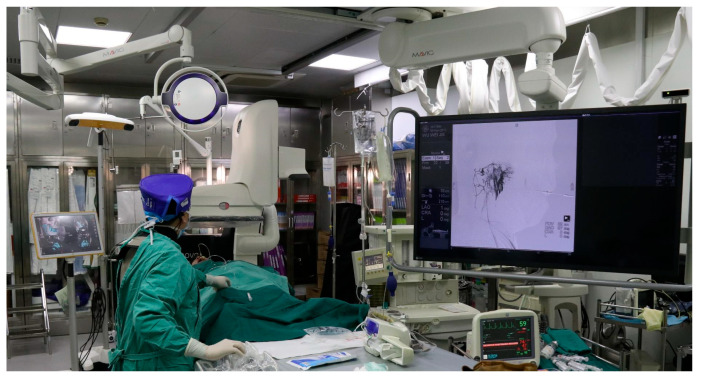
Intraoperative process of real-time MRI combined with optical navigation system. In addition to the MRI scanner, the setup includes a detachable bedside positioning frame that can be mounted at variable locations along the examination table to accommodate different treatment sites; an infrared tracking camera that captures reflected light from trackable fiducial markers to compute the real-time spatial relationship among instruments, the patient table, and the MR scanner; and two models of MR-compatible puncture needles provided to suit different lesion depths and access trajectories.
